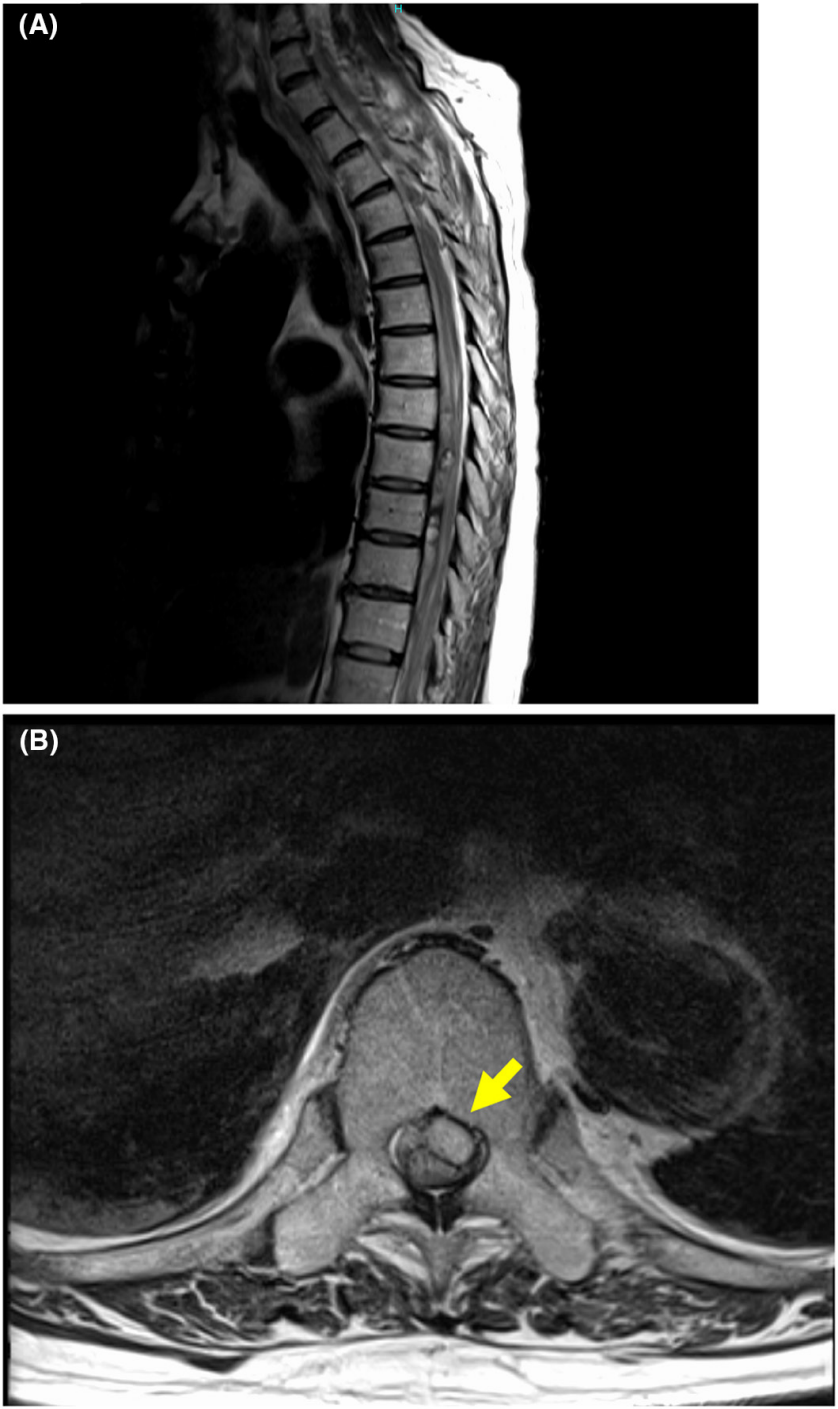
Preoperative MRI. (A) Sagittal T2‐weighted MRI revealed evidence of intradural hematoma from the T7 level to the T10 level. (B) Axial T2‐weighted MRI revealed a hematoma on the ventral side of the spinal cord at the T10 level.
